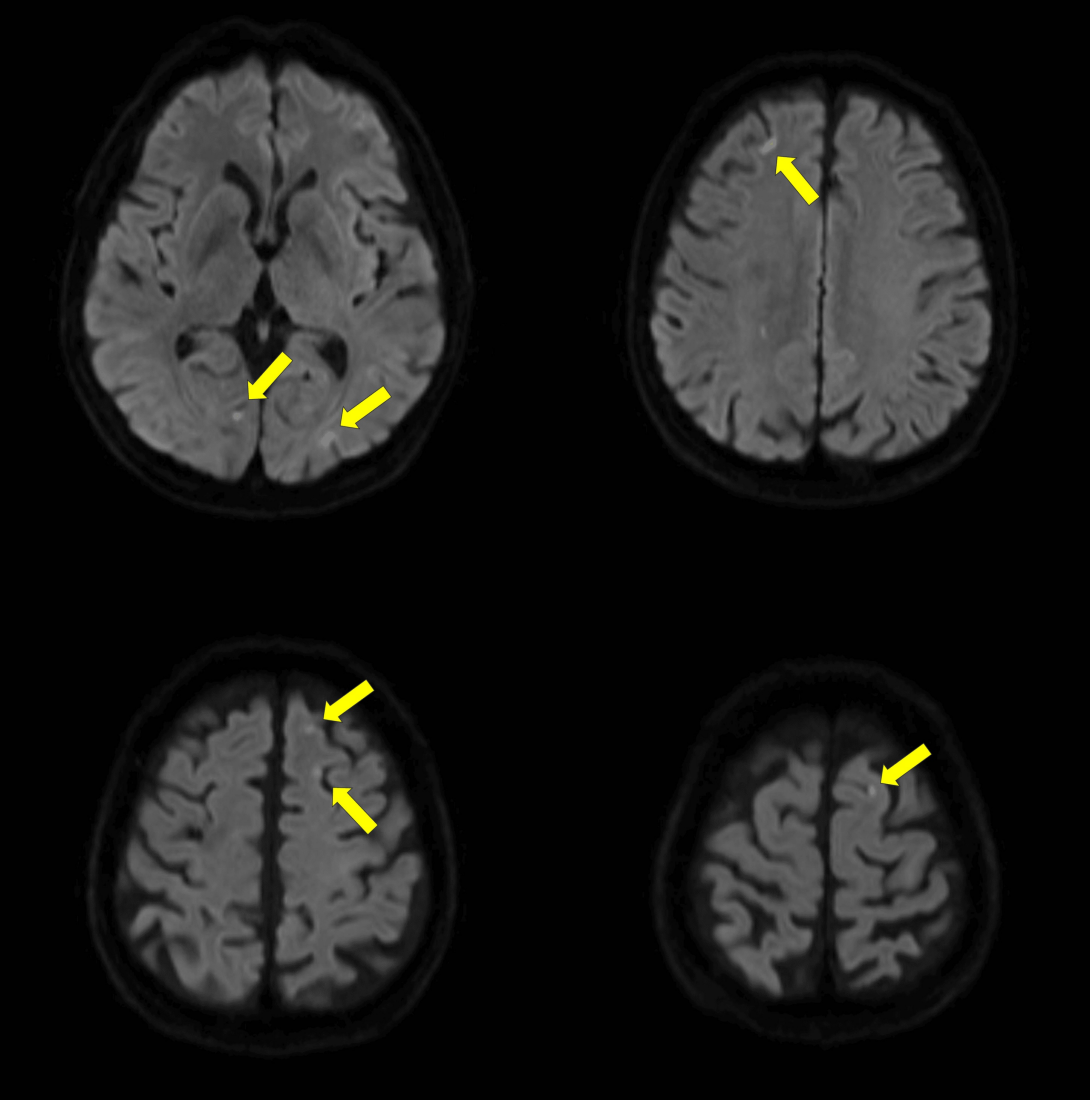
MRI showing bilateral cerebellar infarctions (arrows indicate the lesions).
